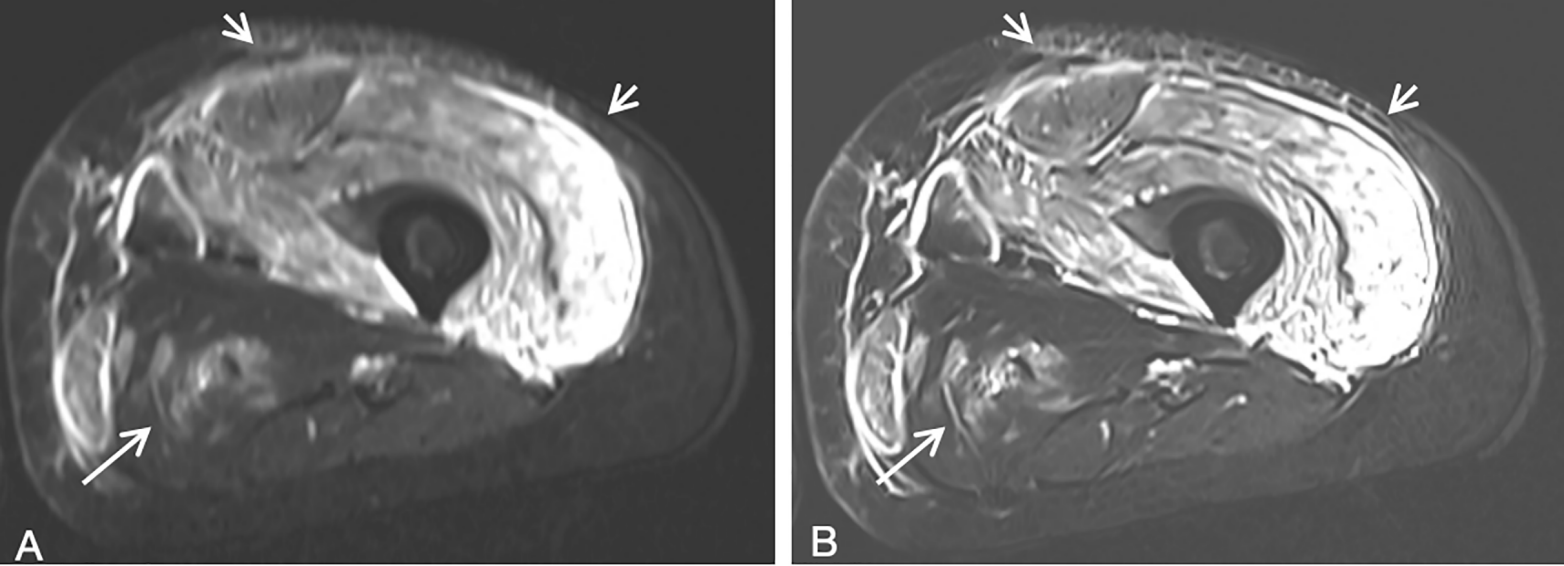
Thigh MRI images of a 30-year-old female patient with dermatomyositis. (A) Standard TSE. (B) TSE_DL_. Marked high signal intensity of thigh muscles on T2WI were observed, especially the quadriceps femoris. Compared with standard TSE image, subcutaneous fasciitis (short arrows), and fasciitis of left thigh muscles including adductor magnus (long arrow) are more clearly shown on TSE_DL_ image, which serves as a key image marker for differentiating dermatomyositis from polymyositis, both of which shared the common features of proximal skeletal muscle weakness and muscle edema.
